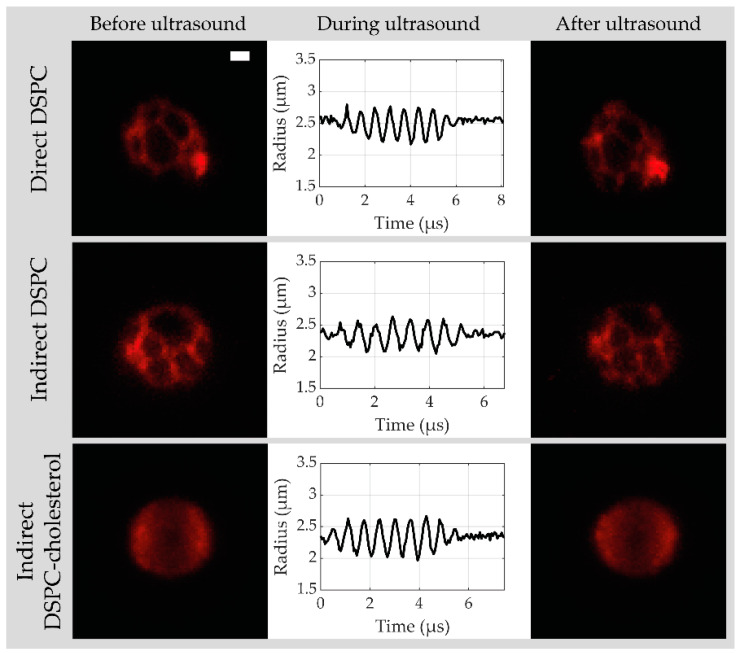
Maximum intensity projections of confocal *z*-stack from direct DSPC, indirect DSPC, and indirect DSPC-cholesterol (12 mol%) microbubbles with the LE phase in red, before and after ultrasound, with the microbubble radius as a function of time obtained from the Brandaris 128 ultra-high-speed recordings during ultrasound (50 kPa peak negative pressure (PNP), 1.6 MHz). Scale bar is 1 µm and applies to all images.
